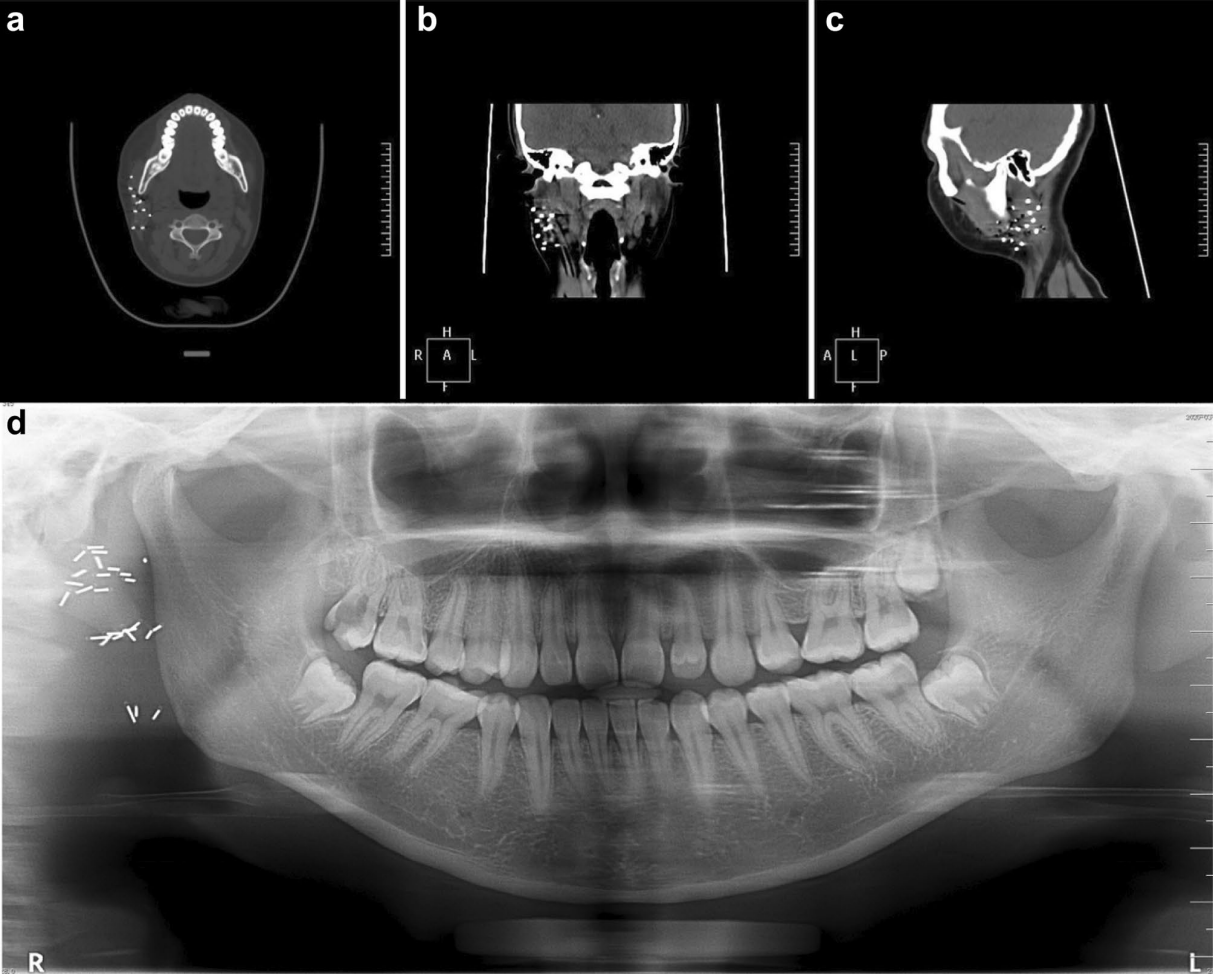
A typical postoperative implantation of I^125^ radioactive particles. (a) Horizontal plane; (b) coronal plane; (c) sagittal plane; (d) panoramic radiography.
